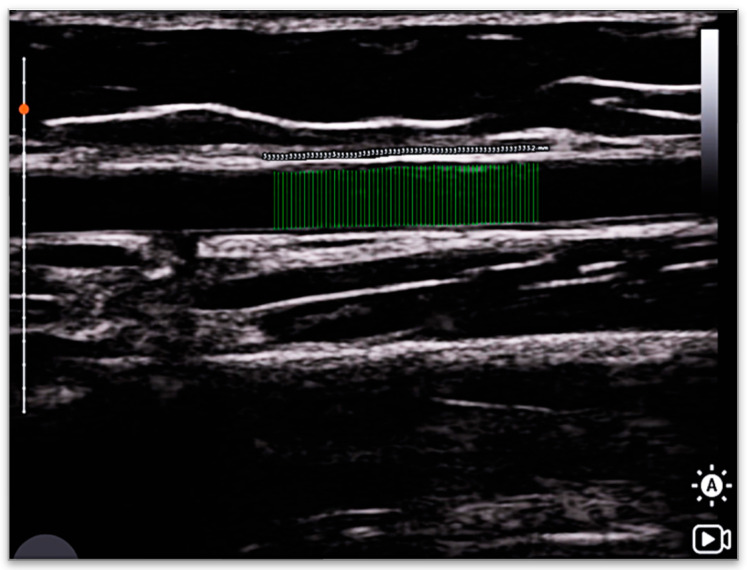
Representative output of the AI-assisted handheld ultrasound (handheld ultrasound, HHUS) vascular image analysis system, showing the results displayed after image upload and completion of the automated analysis.
